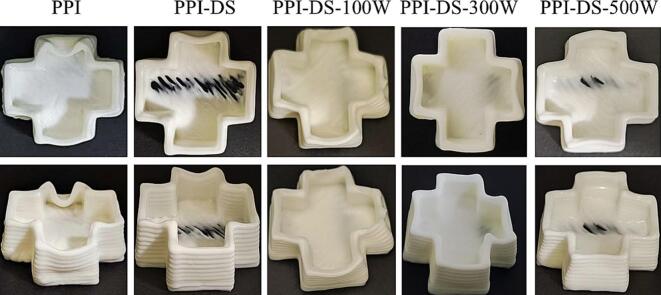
3D printability of HIPEs stabilized by PPI and PPI-DS with different ultrasound powers.
